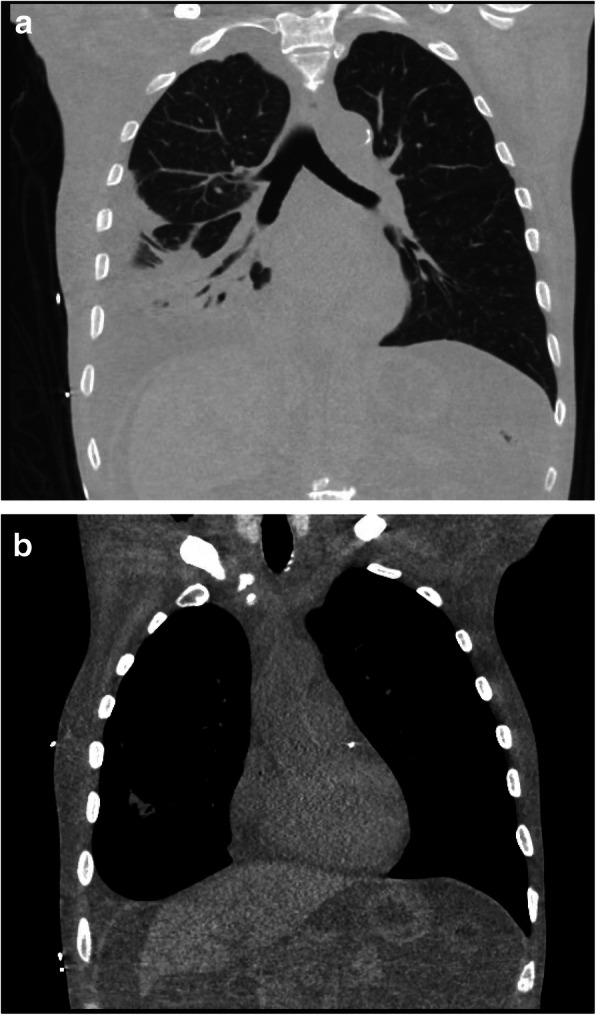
a and b Chest computed tomography with contrast showing large right pleural effusion (a) and large ascites (b)
